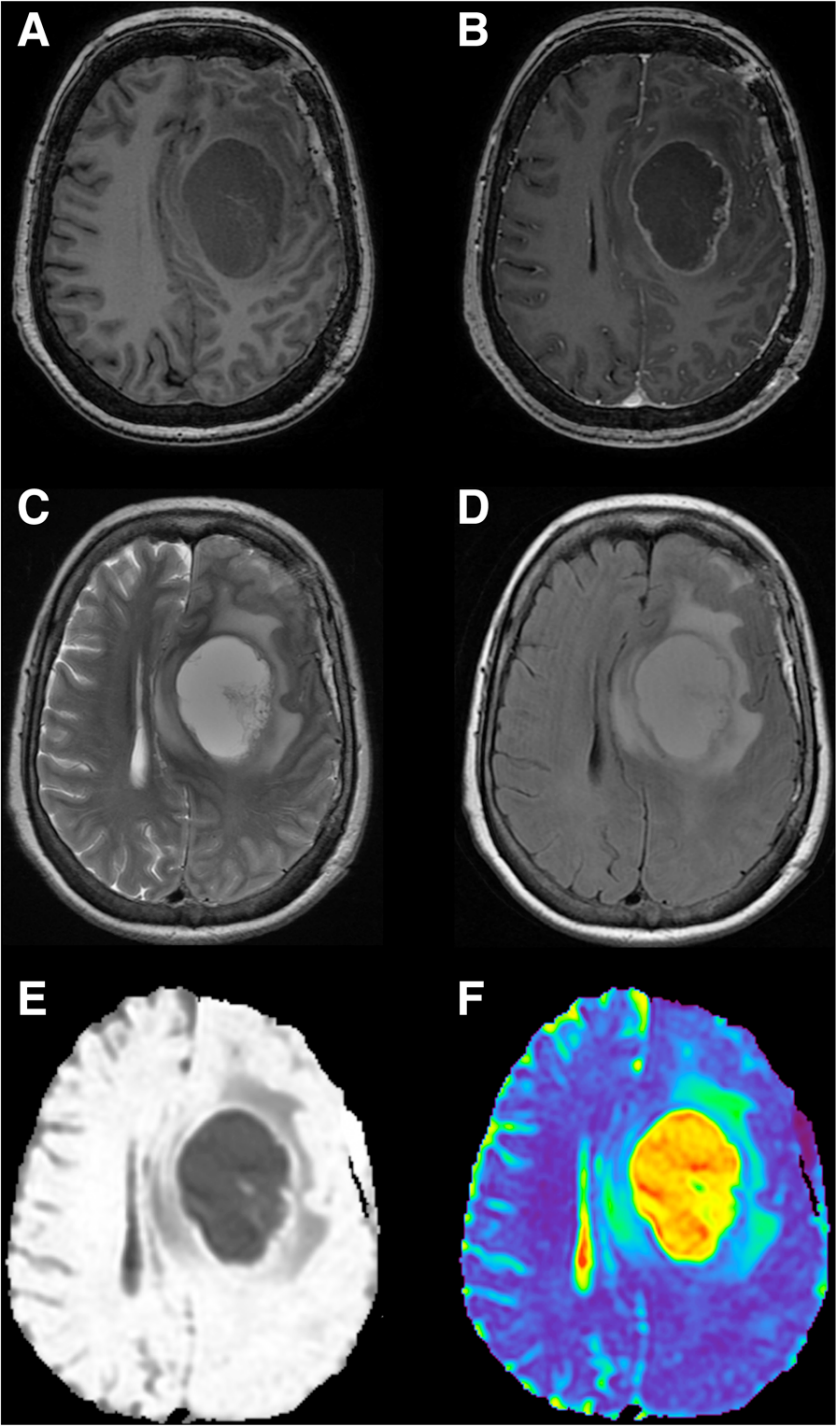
Conventional MR sequences in the axial plane for the assessment of GB. a T_1_-weighted imaging. b Post-gadolinium T_1_-weighted imaging. c T_2_- weighted imaging. d Fluid-attenuated inversion recovery (FLAIR). e Diffusion-weighted imaging (DWI). f DWI-derived mapping with the calculation of apparent diffusion coefficient values
